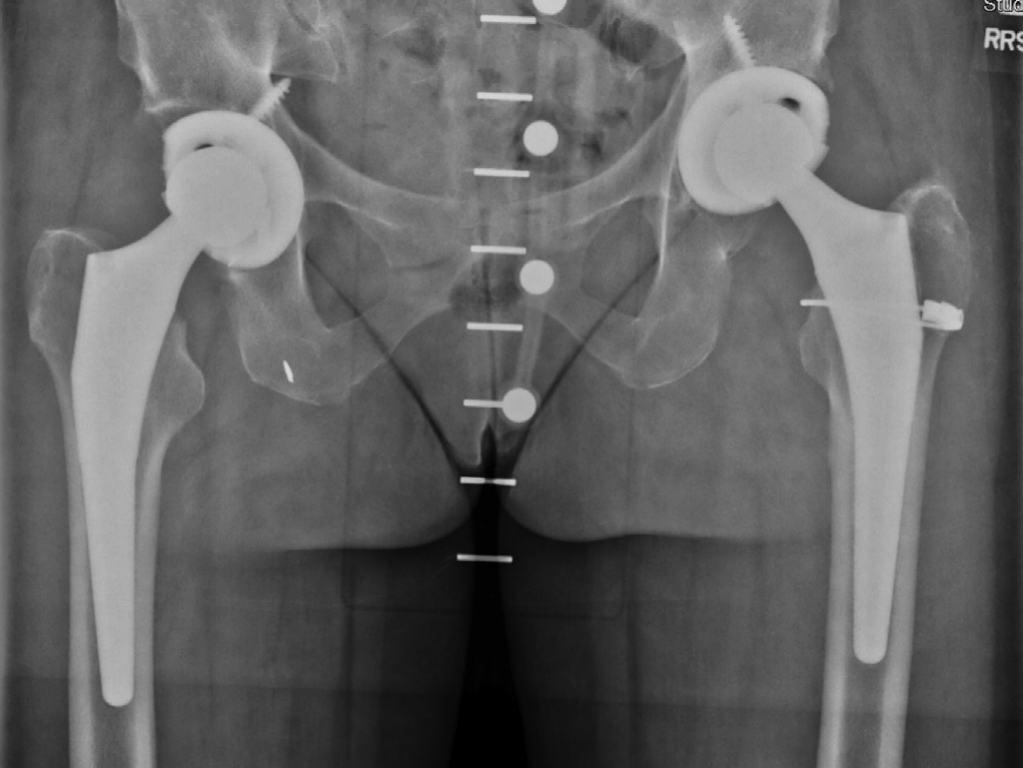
Postoperative pelvic radiograph after staged bilateral total hip arthroplasty, July 2013.
